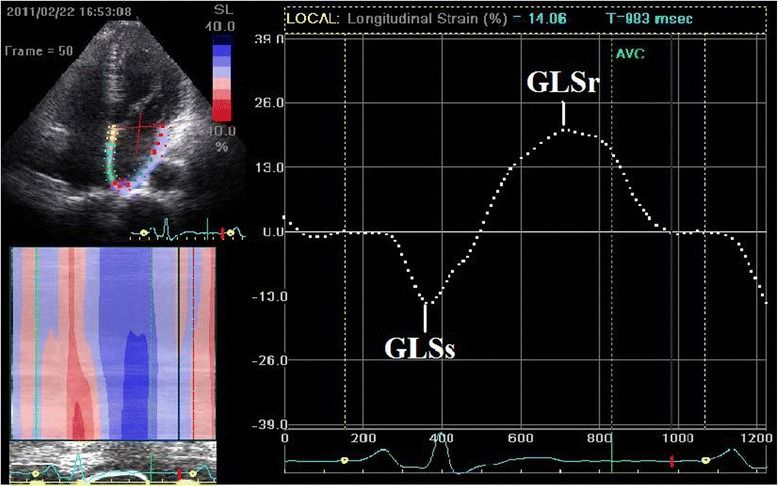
Measurement of global longitudinal left atrial strain from an apical four-chamber view. The white dashed curve represents the global longitudinal atrial strain along the cardiac cycle. GLSs = global left atrial peak negative strain during atrial systole. GLSr = global left atrial peak positive strain during ventricle systole. AVC = aortic valve closure
